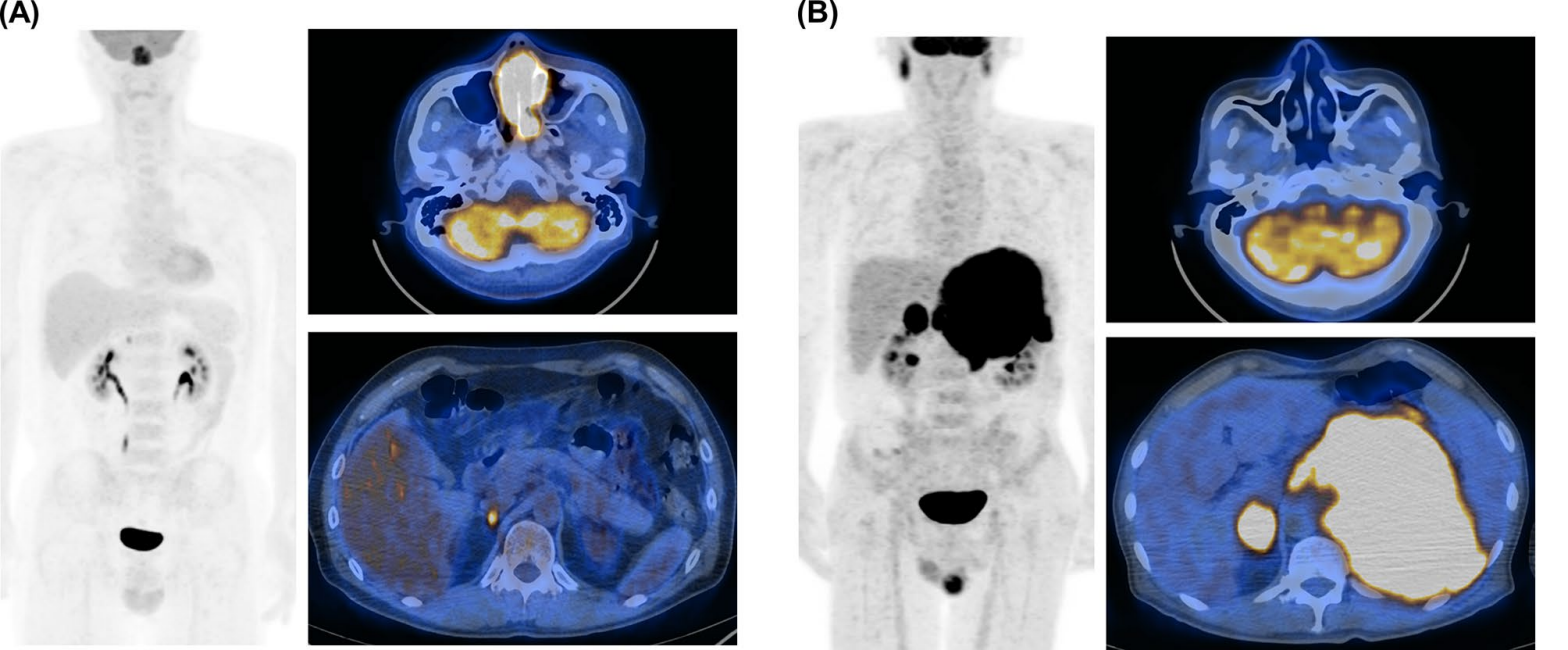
Representative PET/CT images of ENKTL patients. (A) Maximum intensity projection (left) and transaxial fusion PET/CT (right) images of a 50-year-old male showing tumor with highest SUVmax in the nasal cavity (MTV = 43, TLG = 318). The patient had a single IS-favoring PET pattern (small adrenal FDG lesion) and was confirmed to have IE-A subtype. (B) Maximum intensity projection (left) and transaxial fusion PET/CT (right) images of a 56-year-old male with tumor lesions in adrenal glands and testis. The patient had normal nasal cavity uptake but high MTV and TLG on adrenal lesions. This case had all four IS-favoring PET patterns and was confirmed to have IS subtype.
